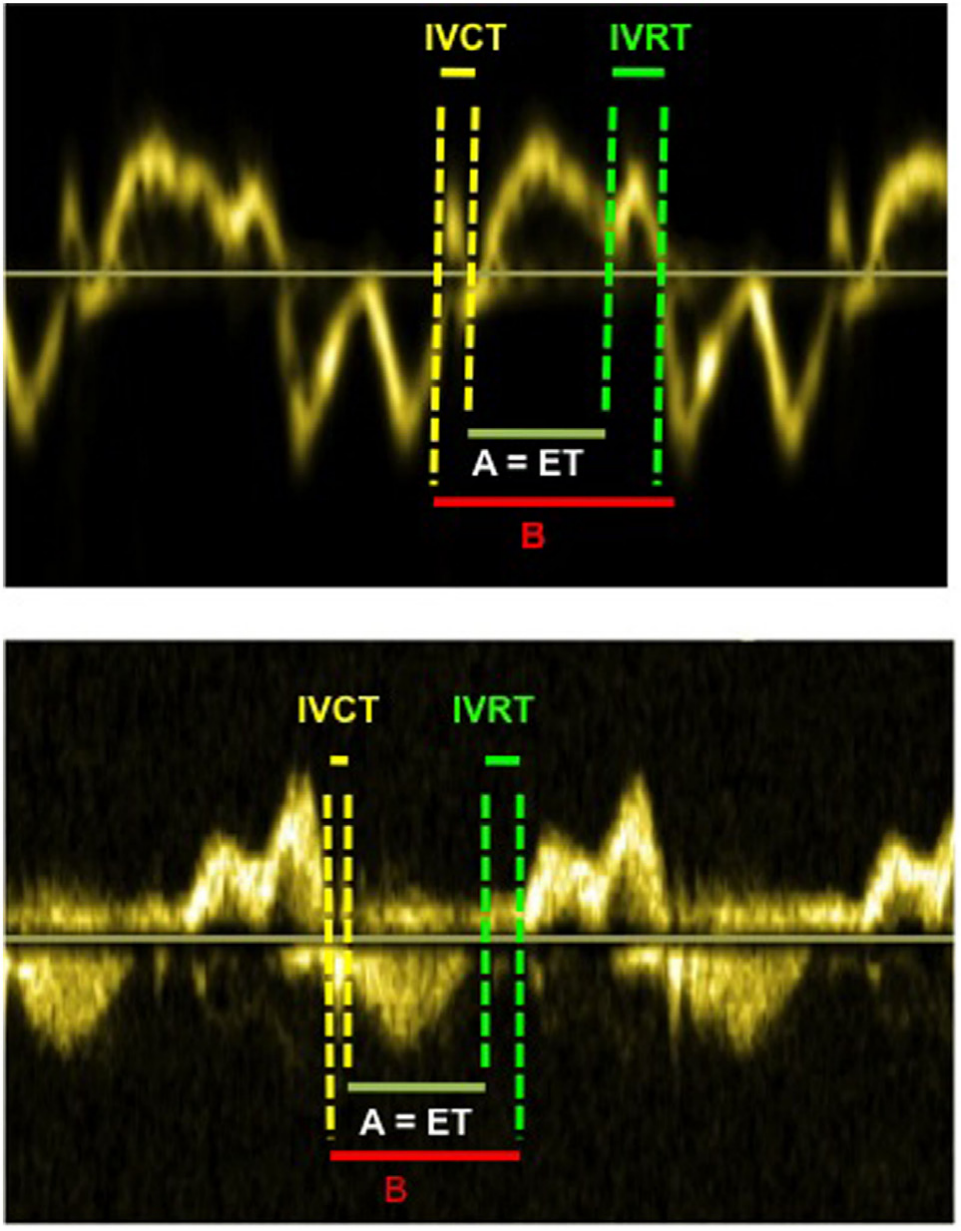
Myocardial performance index (=MPI or Tei index) obtained from tissue Doppler (above image) and from Doppler (below image). The MPI is calculated as the (IVCT + IVRT)/ET or (B − A)/A. The MPI allows evaluation of systolic and diastolic ventricular function. Abbreviations: ET, ejection time; IVCT, isovolumetric contraction time; IVRT, isovolumetric relaxation time.
